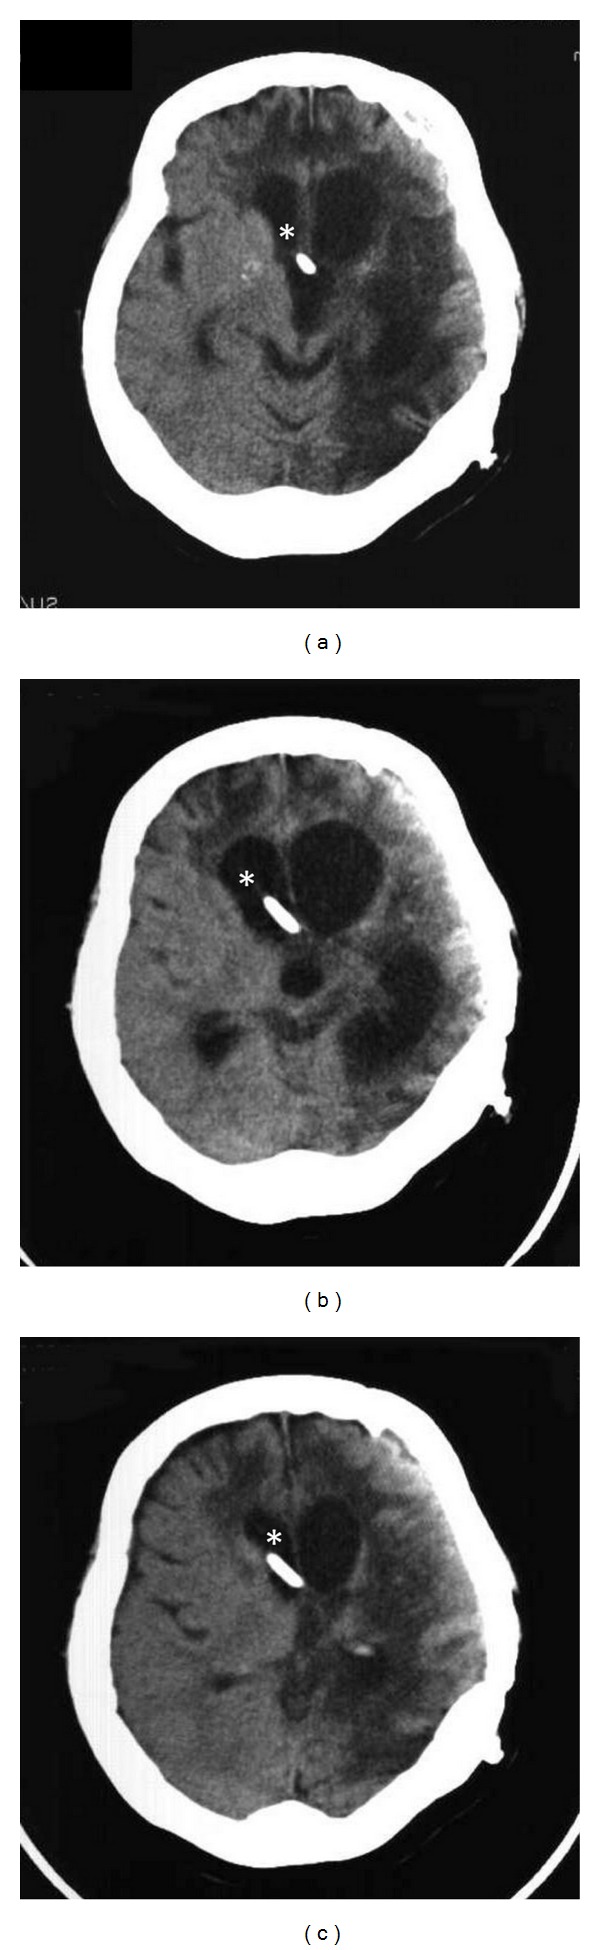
Head computer tomographic (CT) scan image that was performed at the time of admission (a) indicating previous destructive changes in the brain due to damage from the prior subarachnoid hemorrhage and vasospasms with mild hydrocephalus. Head CT scan image showing acute deterioration (b) indicating progression of the hydrocephalus. Head CT scan image after the ventriculoperitoneal (VP) shunt revision (c) showing improvement of the hydrocephalus. In each head CT scan image ((a), (b), or (c)), the VP shunt tube can be observed (∗).
